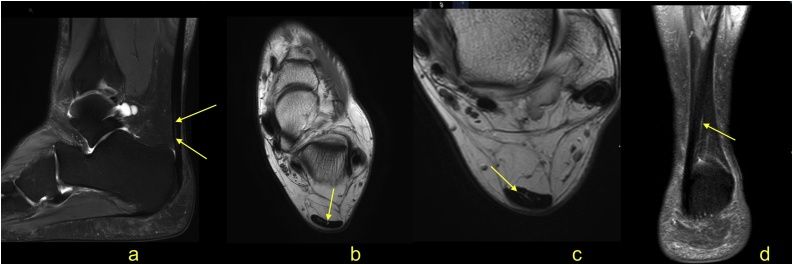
Normal Achilles tendon. A 32-year-old patient with clinical suspicion of a ganglion. MRI of the ankle (a-d) showed the higher signal within the Achilles tendon (arrows), which corresponds to the loose connective tissue between the subtendons. a - sagittal T2-weighted FS; b and c - axial section PD-weighted; d - coronal section PD FS.
